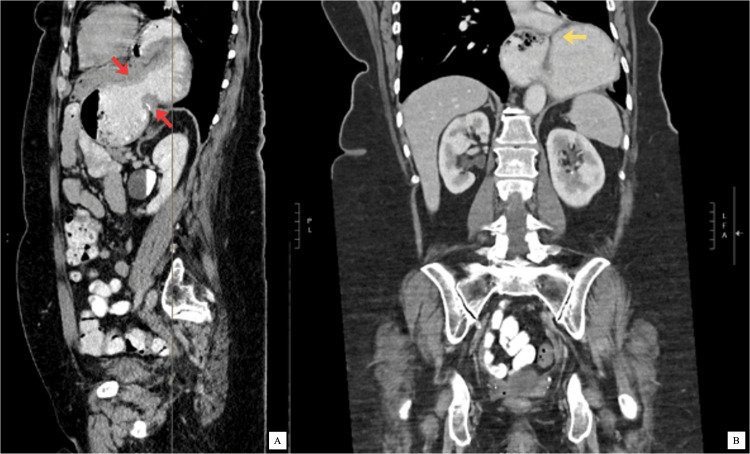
Case 1: Sagittal (A) and coronal (B) views of a CT abdomen/pelvis demonstrating a large hiatal hernia with changes consistent with the patient's history of fundoplication. Red arrows (A): Both point to the herniated stomach above the diaphragm - the upper arrow is on the fundoplication wrap (the heterogeneous/mottled density from the wrapped fundus), while the lower arrow is on the herniated gastric body/hernia sac itself. Yellow arrow (B): Same structure, coronal view - pointing at the wrap/herniated stomach sitting above the diaphragm on the left side. CT: computed tomography
